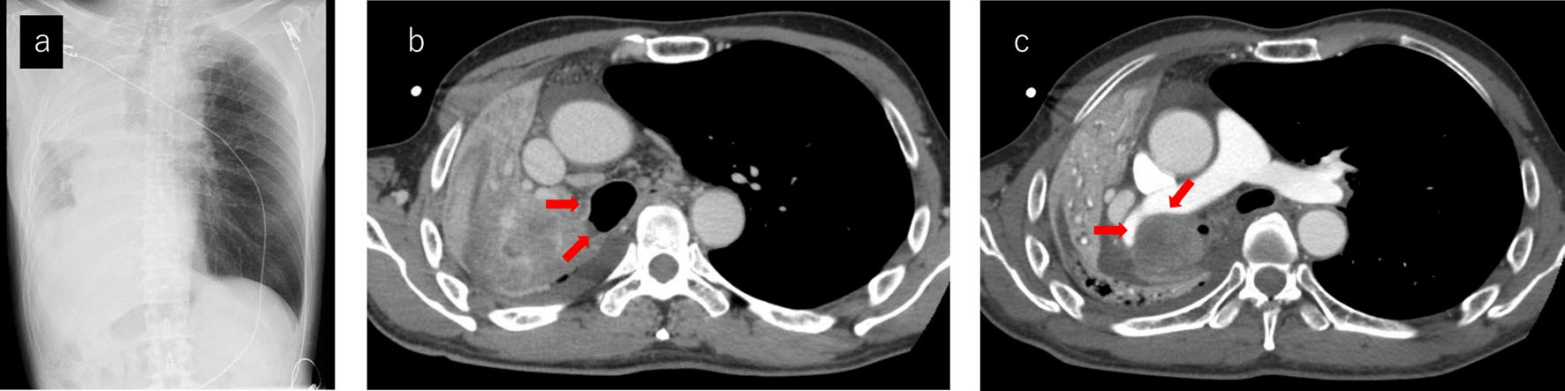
a–c Chest X-ray and CT at the first admission. a Atelectasis of the right lung. b Right main bronchus invasion (red arrows). c Right pulmonary artery invasion (red arrows)
